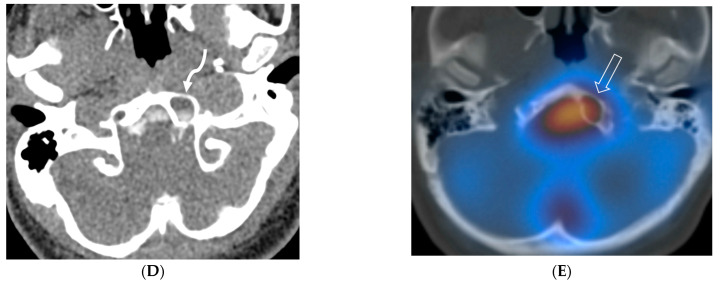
Sagittal T1 (A), post axial T1 (B,C), axial CT myelogram (D) and technetium 99 m DTPA SPECT-CT (E): 16-year-old with Gorham’s disease. There is cerebellar tonsillar herniation and decrease in prepontine cisterns (white arrows). Significant increase in the size of the venous sinuses (dashed arrow). Diffuse pachymeningeal enhancement is seen (black arrows). Cystic-appearing foci at the skull base are in keeping with lymphangiomatosis with contrast pooling into the lytic lesion (curved arrow). Abnormal radiotracer extravasation in the left clival region correlating with lytic lesion (open arrow). Features are in keeping with intracranial hypotension secondary to CSF leak.
